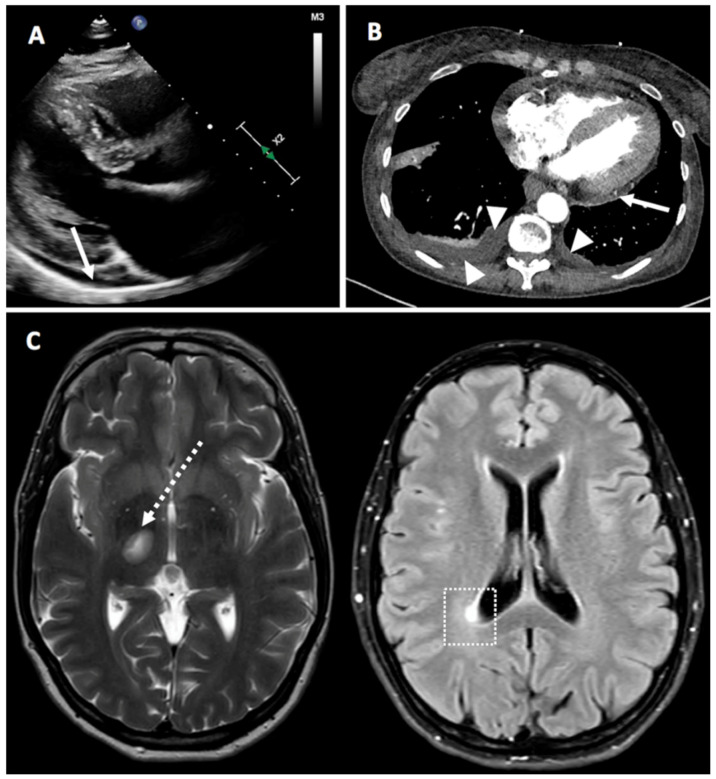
Panel (A) (Case 4)—Parasternal, long axis view from a 2-D echocardiogram showing a small-sized posterior pericardial effusion (white arrow). Panel (B) (Case 27)—Computerized tomography scan demonstrating posterior pericardial effusion (white arrow) and bilateral pleural effusion (white arrowheads). Panel (C) (Case 7)—Magnetic resonance imaging of the brain showing demyelinating lesions in the left mesencephalic (dotted white arrow) and in the right peri-ventricular occipital white matter (white dotted square).
